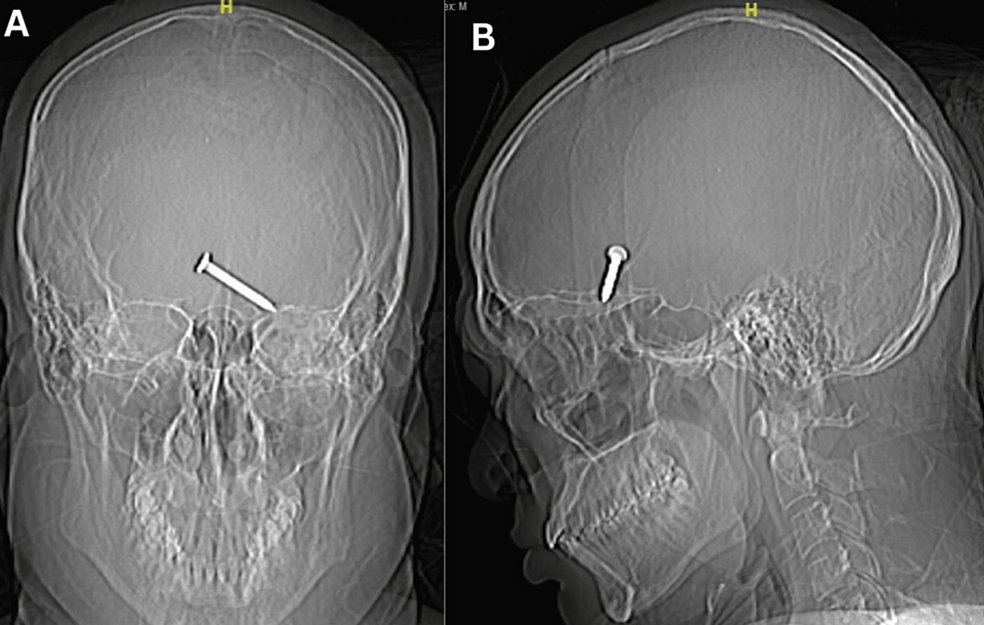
Plain X-ray of the skull, anteroposterior (A) and lateral (B) views, showing a metallic foreign body resembling a nail.
